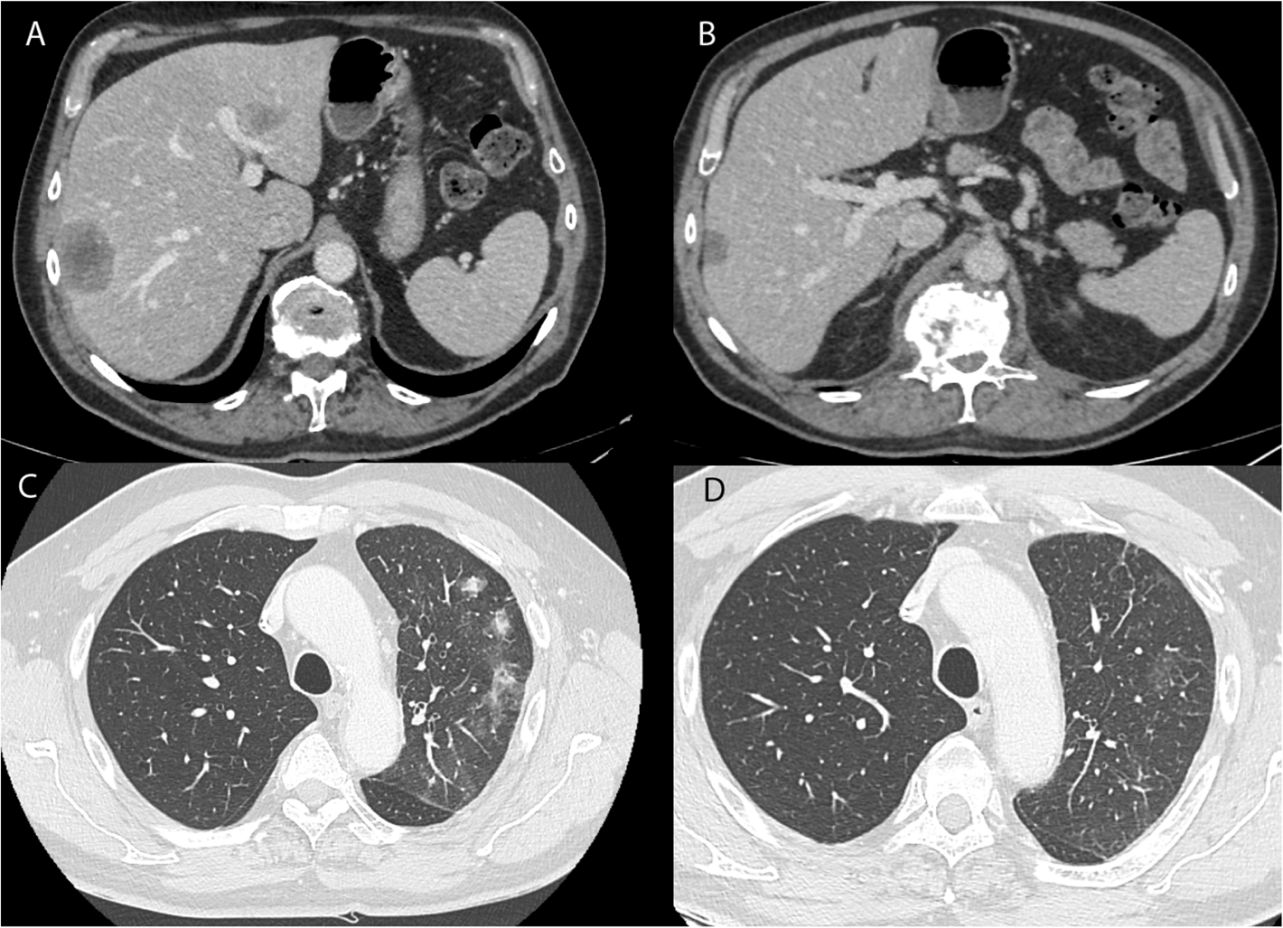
The radiological tumor evolution. a computed tomography (CT) scan at baseline showing hepatic metastases of the lung adenocarcinoma. b CT scan shows a partial response after 8 cycles of immunotherapy. c pulmonary progression on the CT scan at 4 months after the discontinuation of immunotherapy. d CT scan image showing a partial response after 8 cycles of immunotherapy rechallenge
